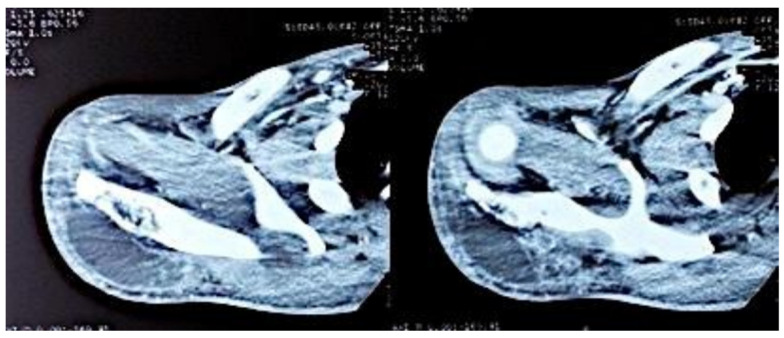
Axial CT sections of the shoulder, showing the lytic lesion of the distal ½ of the right scapular spine.
